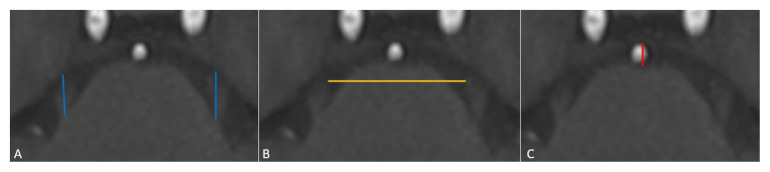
The figure shows the limits used in the evaluation of morphometric length measurements (cm) on T1-weighted MRI scans in a 39-year-old female patient with TN. (A); trigeminal nerve length (blue draws), (B); nerve-to-nerve distance (yellow draw), (C); anterior-posterior prepontine cistern length (red draw).
